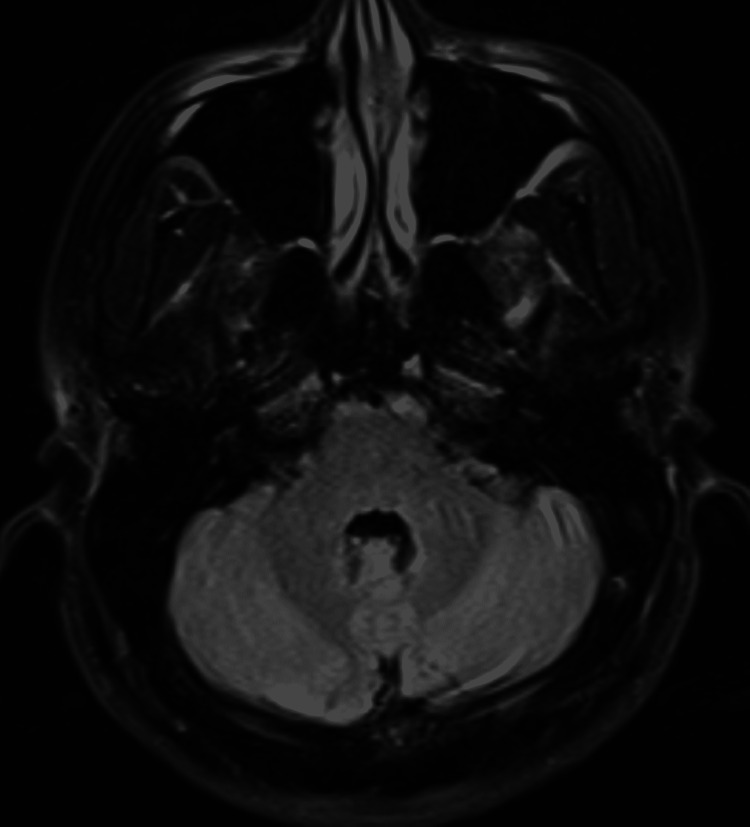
FLAIR of head MRI showing normal posterior fossa.
